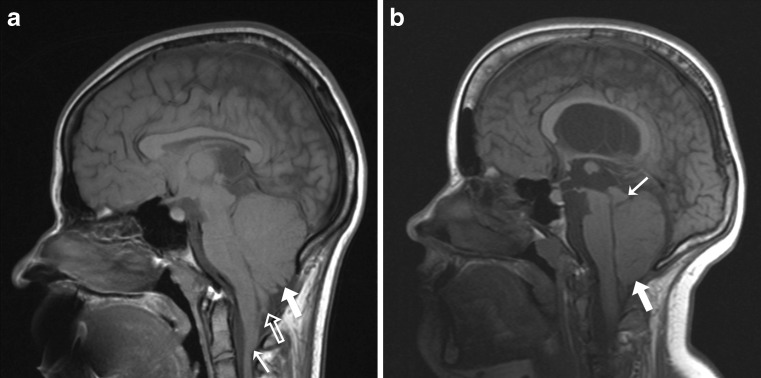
a Sagittal T1-weighted brain MR image in 16-year-old child with open spinal dysraphism. The image shows herniation of the vermis (*large white arrow*), herniation of the tonsil (*large white open arrow*), and medullary kinking (*small white arrow*); b sagittal T1-weighted brain MR image in 12-year-old child with open spinal dysraphism. The image shows herniation of the cerebellum (*large white arrow*). The vermis and tonsil cannot be demarcated from each other. Note the beaked tectum (*small white arrow*) and the hypoplastic tentorium. Also, note the downward displacement of the medulla and pons and the small fourth ventricle in both images
